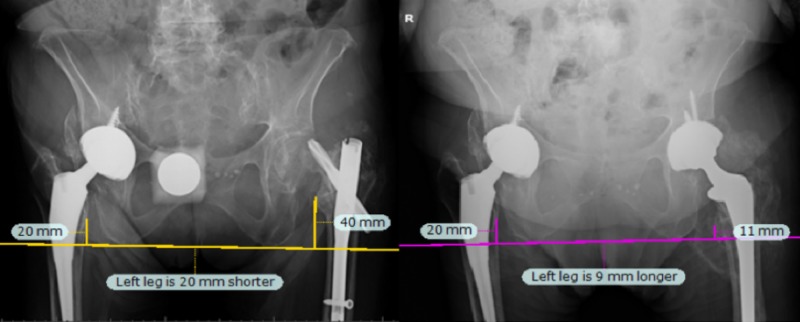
Radiographs for Case 3. Preoperatively (left), the LLD was estimated at 30 mm. During surgery, the operative leg was lengthened by 30 mm, giving equal leg lengths, which was confirmed by navigation and post-operative radiographs (right). LLD: Leg length discrepancy
